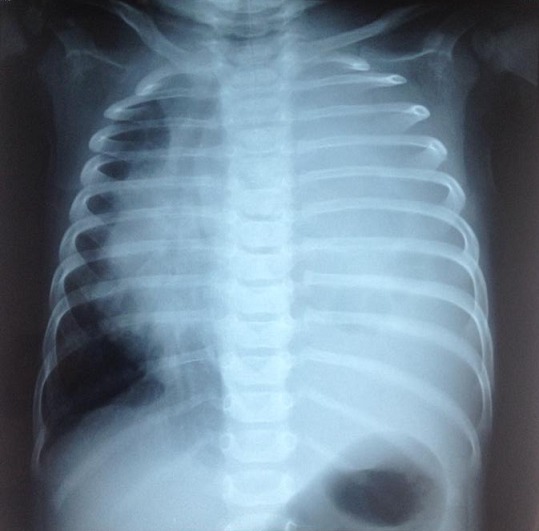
Radiographie thoracique de face montrant une pleurésie à gauche
